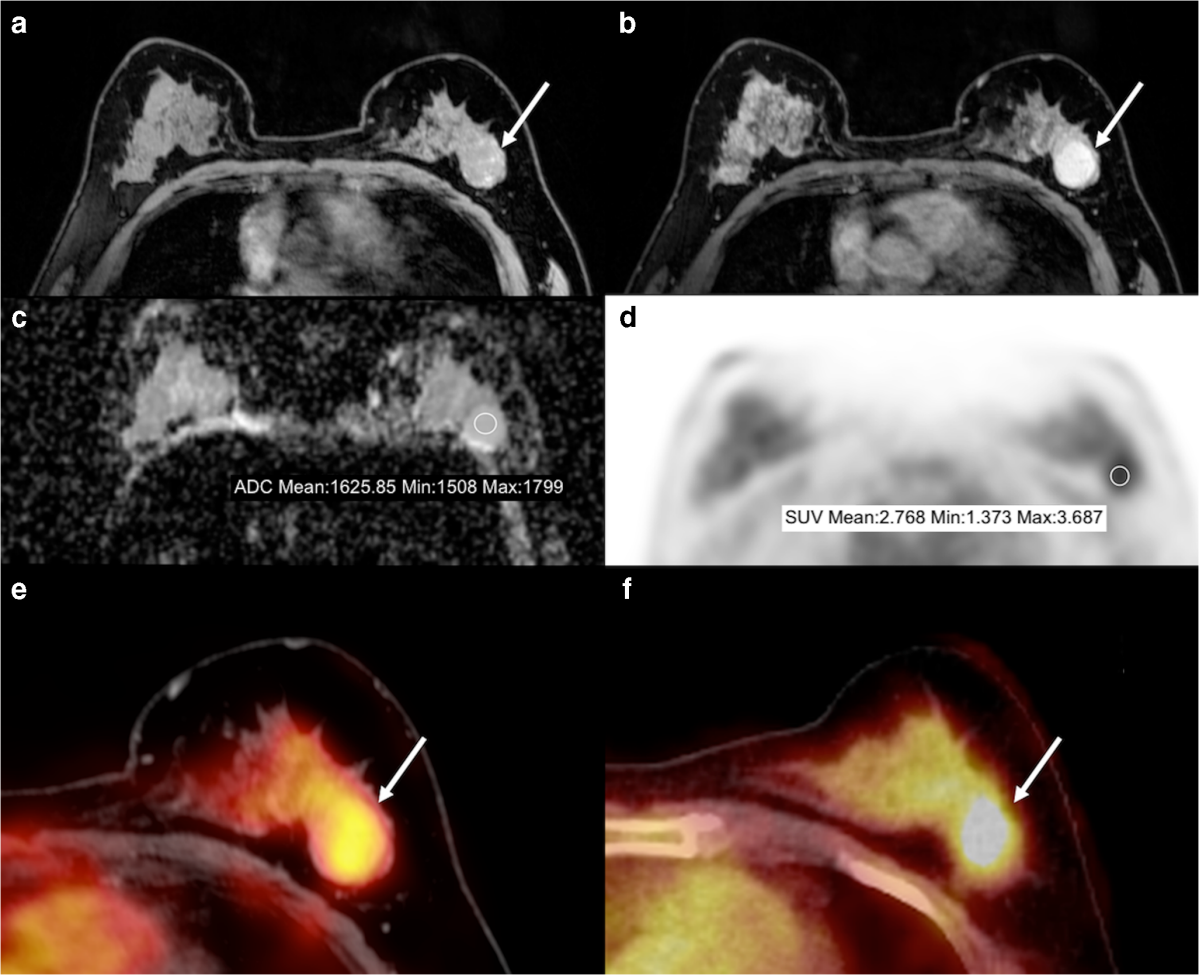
A 55-year-old postmenopausal woman with a fibroadenoma in the left breast presenting as a round, circumscribed mass with homogeneous enhancement on DCE-MRI. The lesion had no hindered diffusivity on DWI with an ADCmean of 1.625 10^−3^ mm^2^/s and was mildly [^18^F]FDG avid on PET with an SUVmax of 3.7. Unenhanced T1-weighted imaging shows heterogeneous fibroglandular tissue (ACR C) of the healthy breast, with moderate BPE on DCE-MRI. ADCmean of the healthy contralateral breast parenchyma is 1.642 10^−3^ mm^2^/s. On [^18^F]FDG PET, BPU of normal breast parenchyma is relatively high with an SUVmax of 3. A unenhanced fat-saturated T1-weighted MRI; B DCE-MRI; C DWI; D [^18^F]FDG PET; E fused [^18^F]FDG PET/MRI; F [^18^F]FDG PET/CT
